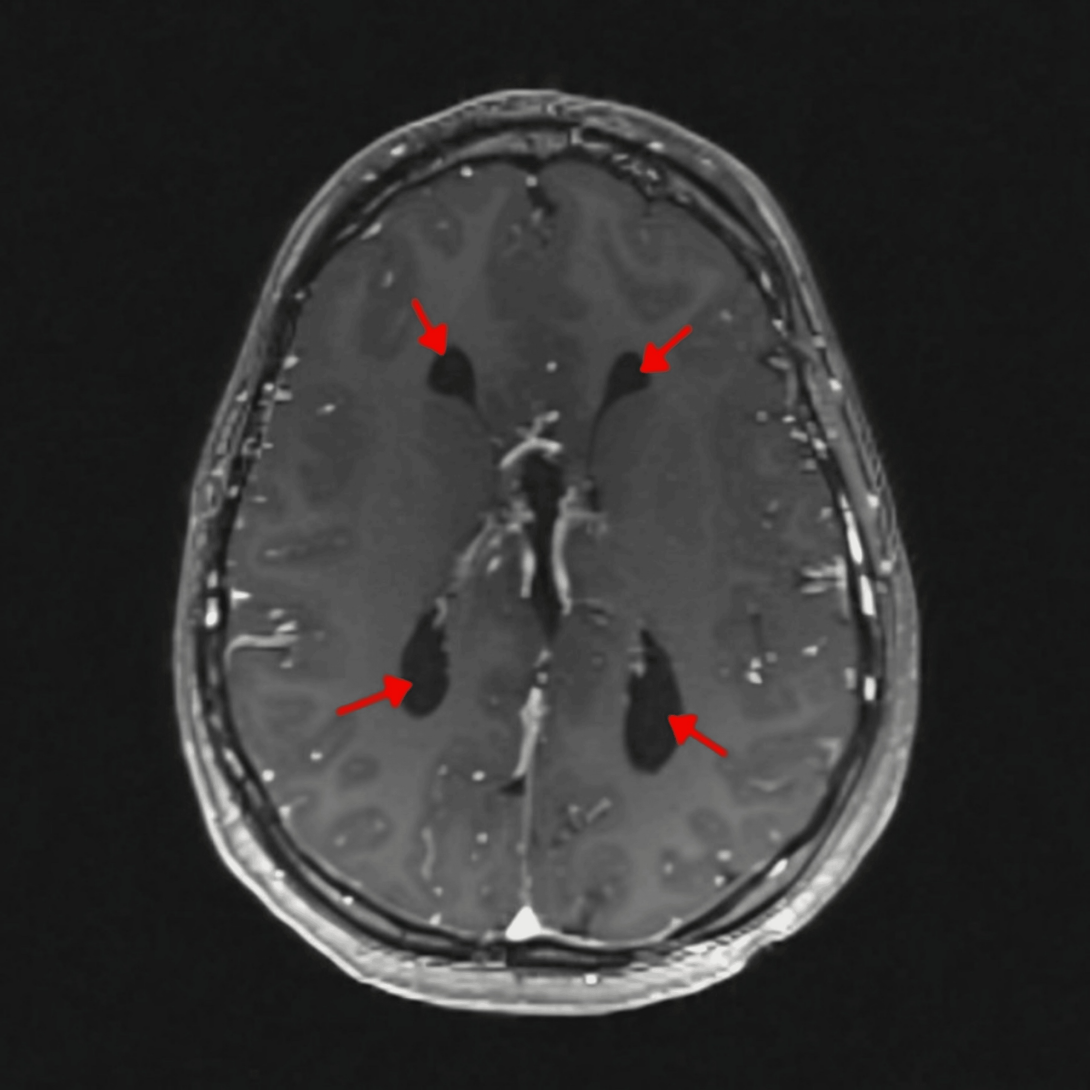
MRI brain shows widely separated lateral ventricles parallel to each other (red arrows), a typical feature of agenesis of the corpus callosum.
